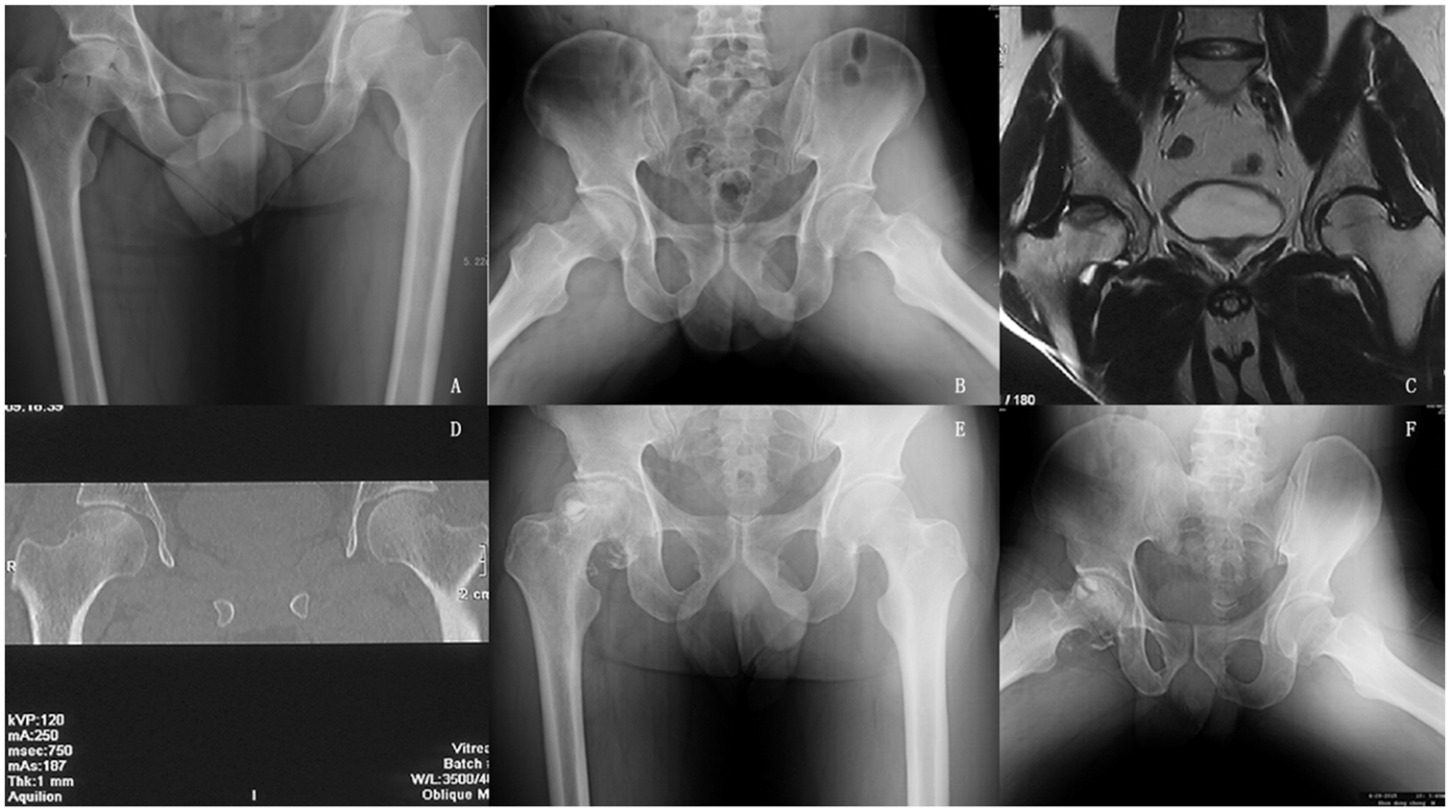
Radiographs of a 46-year-old patient with osteonecrosis of the femoral head of the right side. A) and B) Serial AP and frog lateral radiographs showing ONFH ARCO stage IIIa/CJFH type L2 on the right sides. Subchondral insufficiency fracture can be clearly observed on the frog lateral radiographs. C) and D) Preoperative magnetic resonance image shows that the necrotic lesions involve the lateral pillar. E) and F) Serial AP and frog lateral radiographs taken eight months post operation show a progressive collapse of the femoral head.
